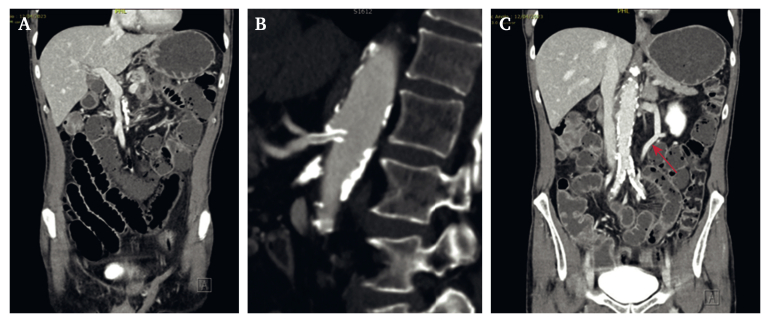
Repeat computed tomography angiography images. A. Ischaemic changes. B. Patent coeliac artery stent. C. Arc of Riolan (indicated with red arrow).
